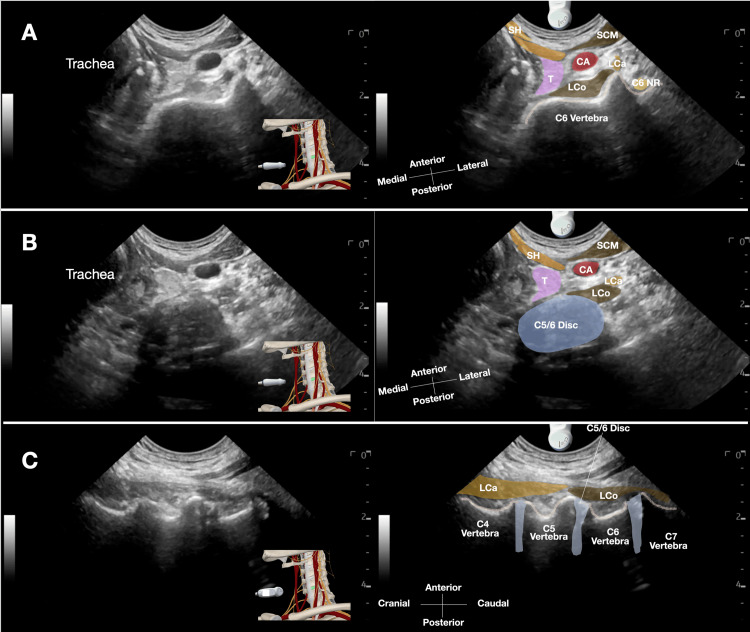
Ultrasound identification of the target cervical disc using short-axis and long-axis views. (A) Short-axis image at the C6 level, demonstrating the C6 vertebral body and C6 nerve root as segmental landmarks.
